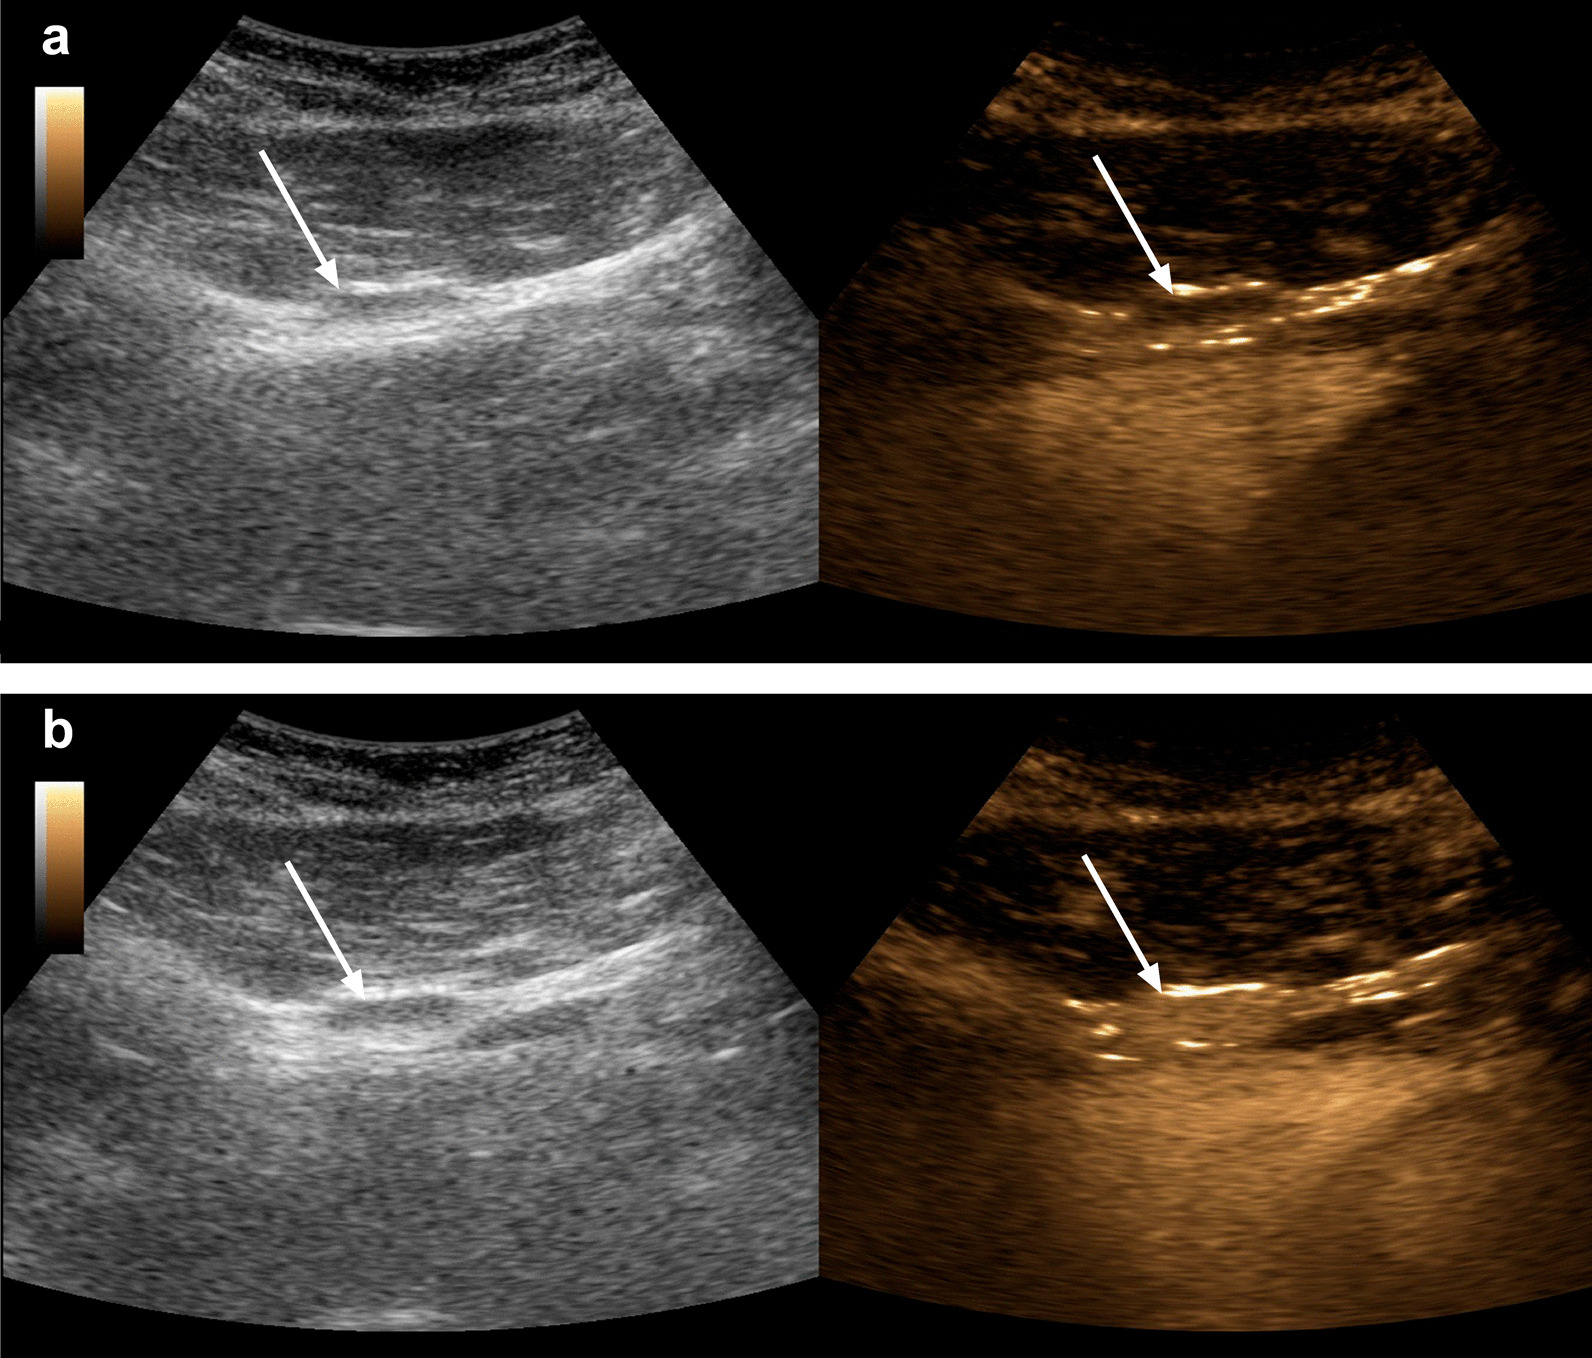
Contrast-enhanced ultrasound of a splenotic lesion between abdominal wall and liver. Images are taken before (a) and during persistent (> 4 minutes) late-phase contrast enhancement typical for splenic tissue (b)
